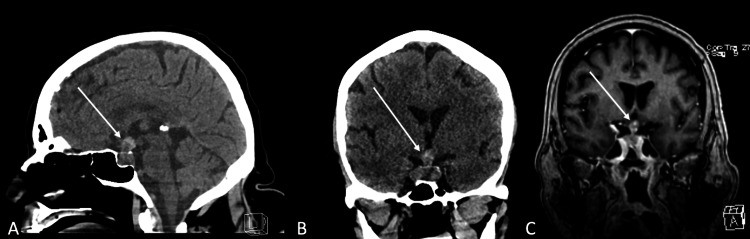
Presentation imaging. A, B: Noncontrast CT scan demonstrating a 2.4 × 1.2 cm lesion in the sella and suprasellar cistern. C: Postcontrast T1-weighted MRI demonstrating a lesion in the sella turcica with heterogeneous signal intensity and enhancement. CT: computed tomography; MRI: magnetic resonance imaging
